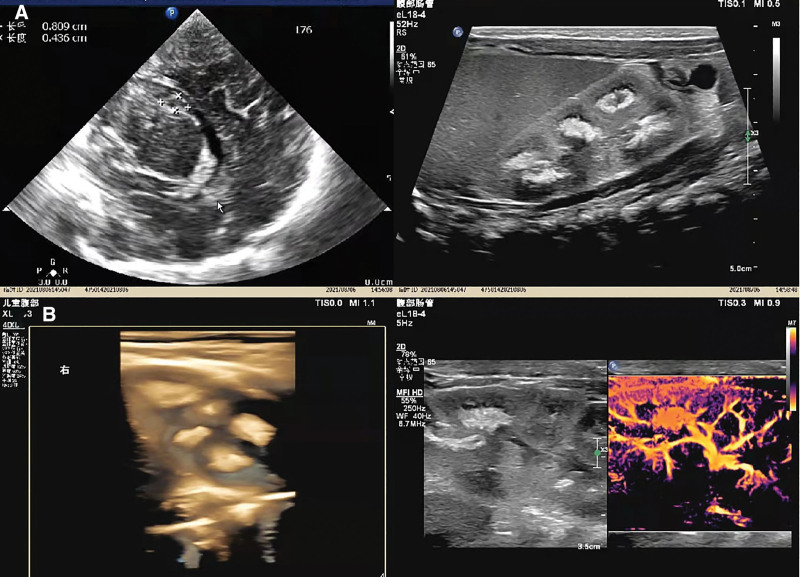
Imagological and other auxiliary examinations. (A) Ultrasonography indicates the absorption period of bilateral subependymal hemorrhage. The sagittal view through the fontanel reveals a hyperechoic area measuring about 0.8 × 0.4 cm in the left subependymal lesion, with a sac-like anechoic zone inside. The bilateral bodies of lateral ventricles are both about 0.2 cm in width. The coronal view indicates sharp anterior horns of the lateral ventricles. The width of the third ventricle measures about 0.3 cm. The thalamus, caudate nucleus and lenticular nucleus are clearly defined. No abnormality was spotted in the echoes of both cerebral hemispheres. (B) Ultrasonography suggests bilateral medullary sponge kidneys. The left kidney measures about 5.0 × 2.2 cm, and the right kidney about 4.2 × 2.2 cm. Homogeneous echoes are detected from the renal cortex. However, diffuse hyperechoic mass was spotted around the collecting system in bilateral medulla kidneys, with no evident posterior shadow observed. There is no noticeable separation of bilateral collecting systems or dilatation of bilateral ureters. CDFI indicates normal blood perfusion in both kidneys. CDFI = color doppler flow imaging.
